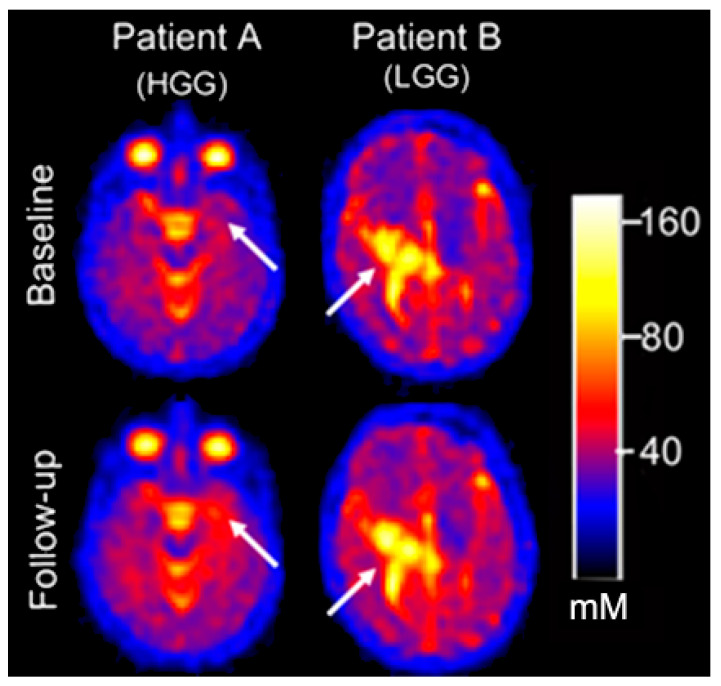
Initial (*top row*) and follow-up (*bottom row*) sodium MRI scans of supratentorial pediatric gliomas with (A) increased total sodium concentration (TSC) corresponding to tumor progression in a supratentorial high-grade glioma (arrows) (HGG), and (B) no change in TSC corresponding to a stable supratentorial low-grade glioma (arrows) (LGG).
